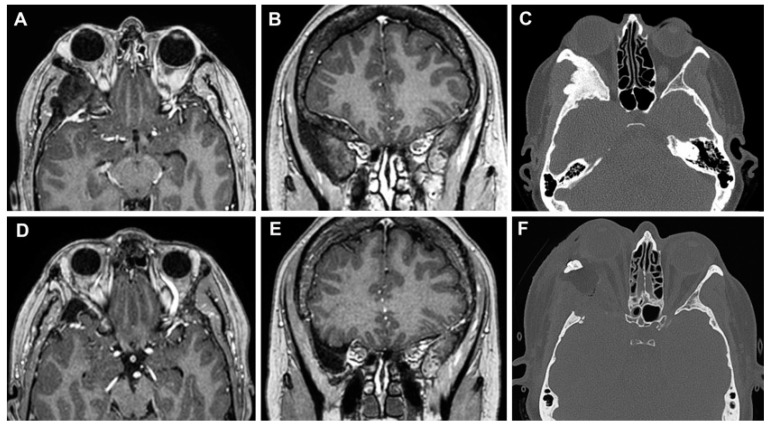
Left sphenoid wing meningioma on preoperative MR images (A,B) and on axial CT scan (C). The post-operative MRI (D,E) and CT scan (F) documented the GTR of the lesion.
